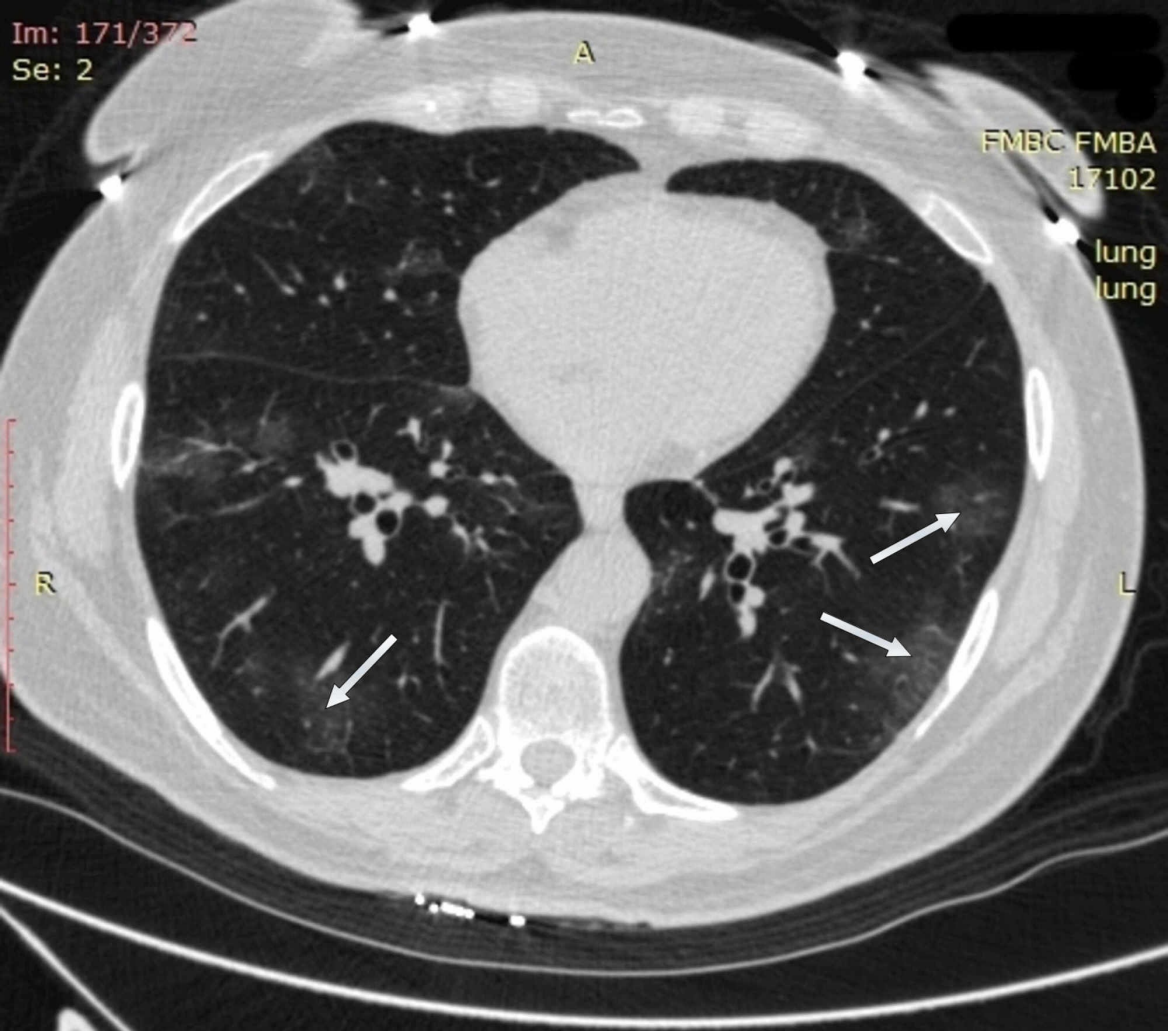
Chest CT imaging of patient 2 on admission Chest CT imaging on admission revealed multiple ground-glass opacities of up to 3 cm in size (white arrows) in the majority of segments of both lungs, predominantly in the lower and middle regions, affecting less than 50% of the lung parenchyma CT: computed tomography
